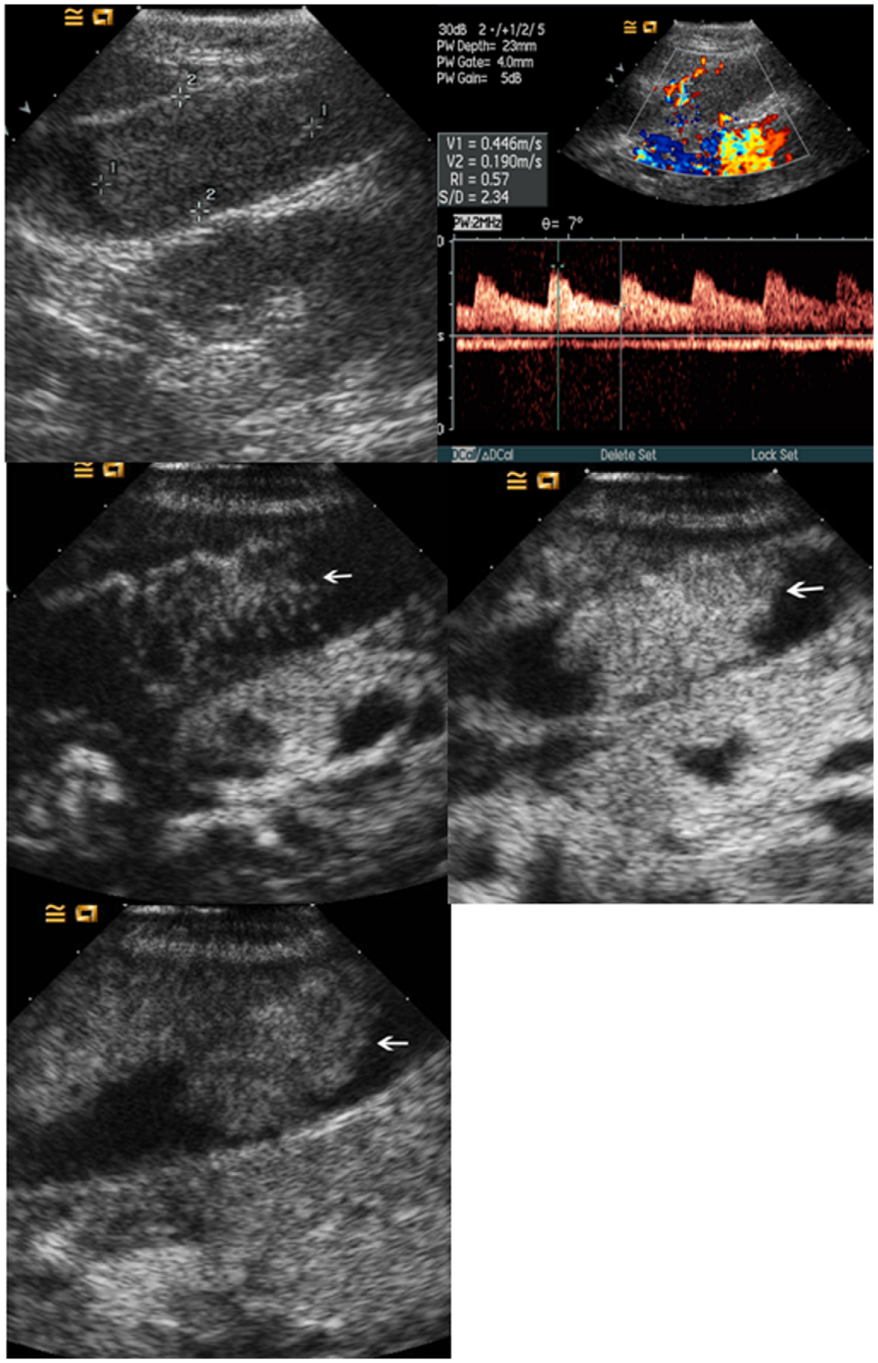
Adenoma in gallbladder. Upper left, conventional ultrasound shows an isoechoic mass (calipers) in the gallbladder; Upper right, pulsatile arterial blood flow is detected in the lesion; Middle left, the lesion (arrow) shows linear blood vessels during the arterial phase; Middle right, the lesion (arrow) shows hyper-enhancement during the arterial phase; Lower left, the lesion (arrow) shows iso-enhancement during the venous phase.
